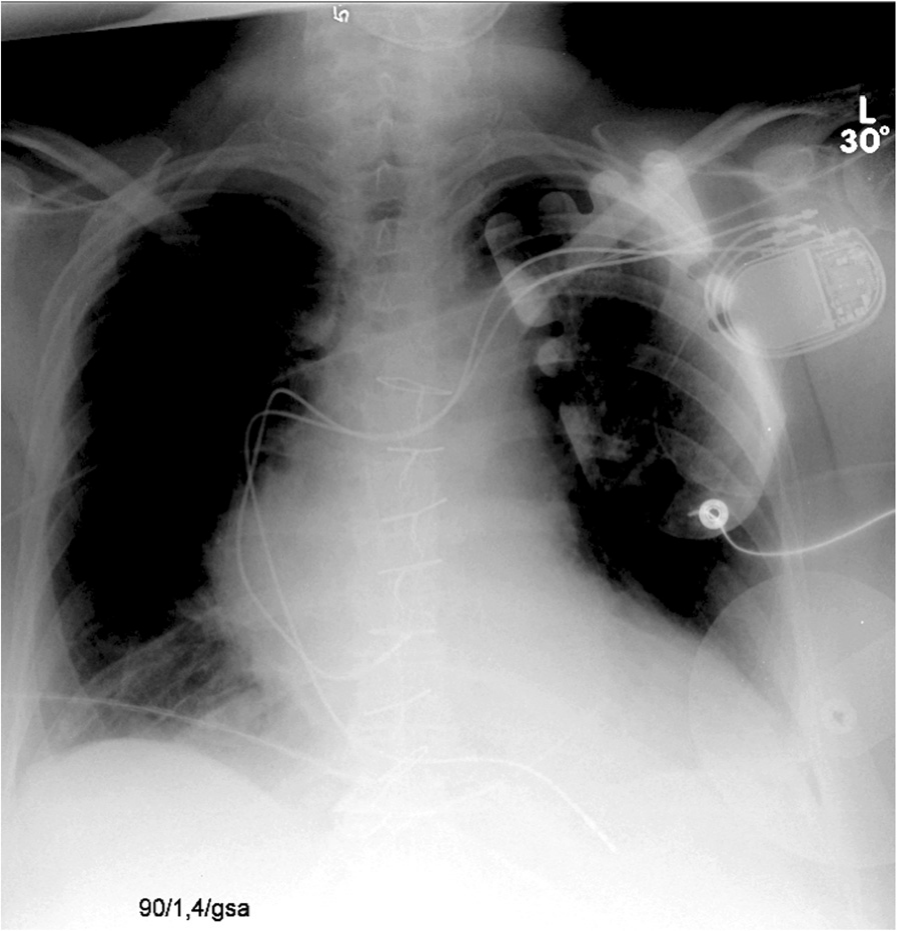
Chest X-ray. Atypical position of the atrial lead.
